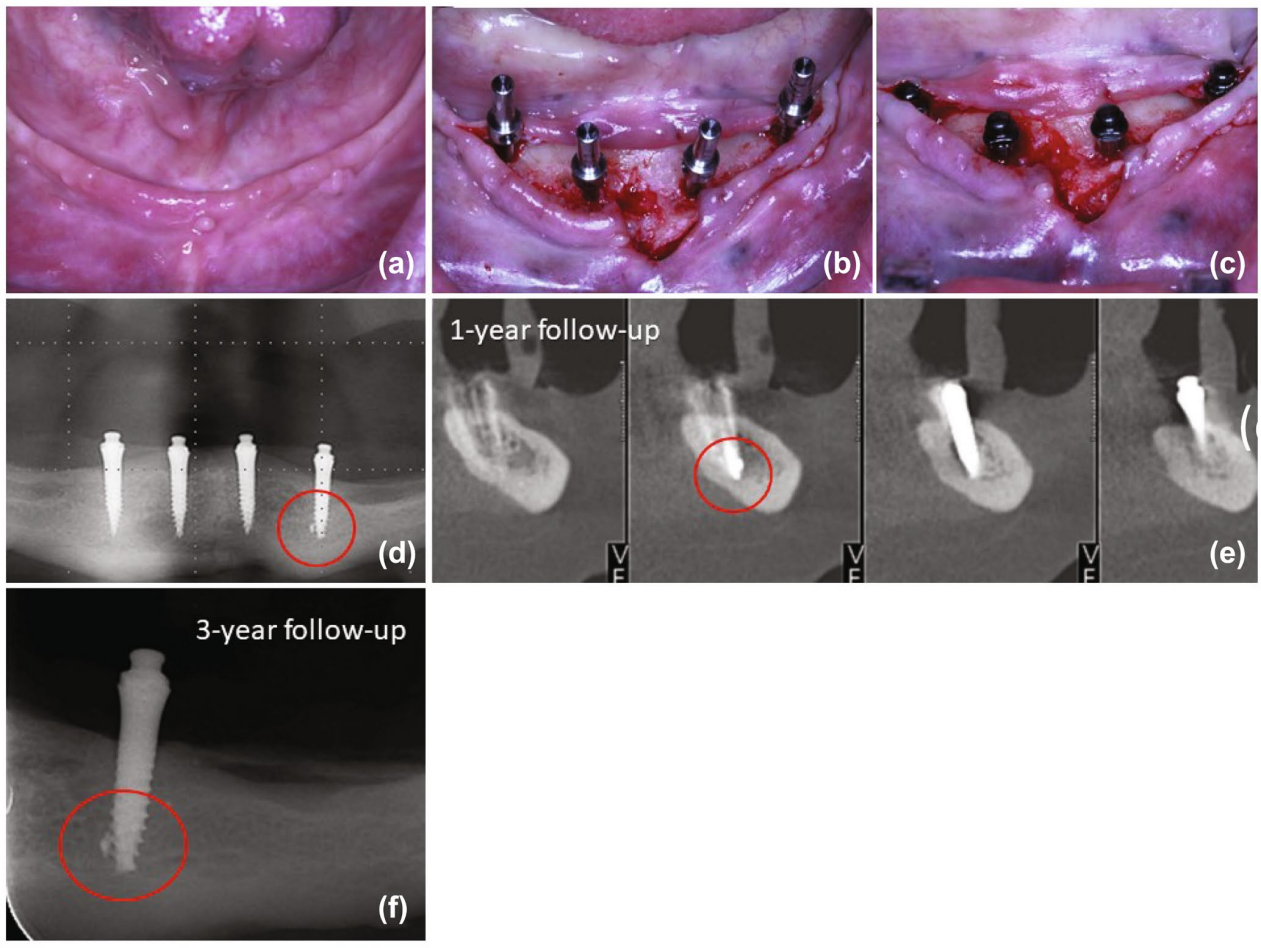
(a) Presurgical view; (b) Flapped surgery view after bone site preparation and paralleling posts in position; (c) Final aspect of the mini implant placement; (d) Panoramic radiograph at 6‐week follow‐up showing the fractured fragment close to the left lateral implant; (e) CBCT at 1‐year follow‐up showing the satisfactory osseointegration of the implant and the fractured fragment; (f) Periapical radiograph of the lateral left implant at the 3‐year follow‐up evidencing the absence of abnormalities.
